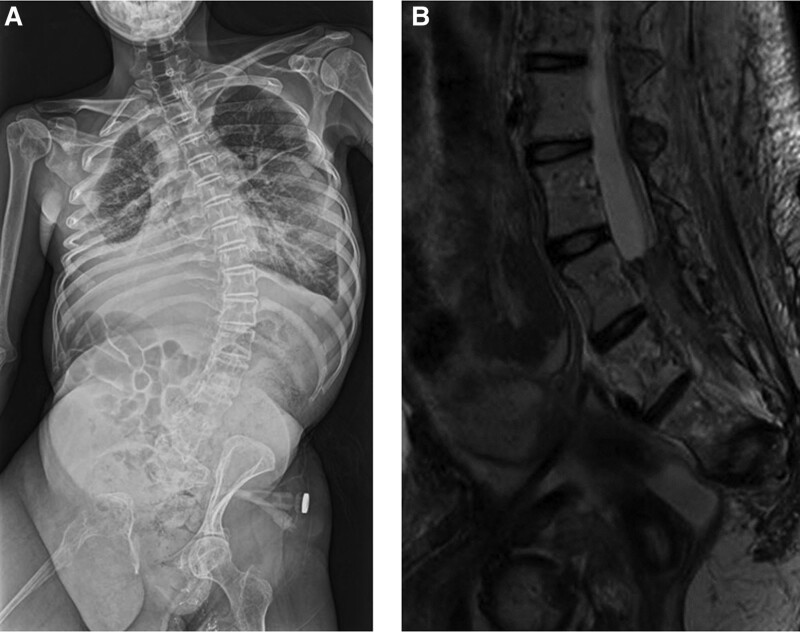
The whole-body radiograph shows massive osteolysis in the right femur, pelvic bone, sacrum, rib, and vertebrae (A). T2-weighted magnetic resonance imaging showing a low-iso intensity area of suspected hematoma from L3 to the caudal region (B).
